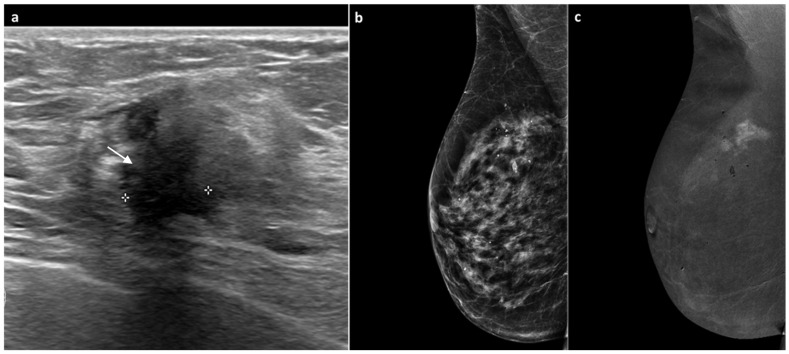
A 77-year-old woman, previously treated for DCIS of the right breast with partial mastectomy 11 years earlier with tenderness at the surgical scar and increased breast consistency. Ultrasound revealed heterogeneous echotexture of the surgical scar with a maximal thickness of 1 cm (white arrow, (a)). Mammography showed no significant changes or suspicious findings (BI-RADS 3) (b). Subsequent CEM demonstrated a marked, intense, multilobulated mass-type enhancement measuring approximately 1.8 cm (c). An ultrasound-guided biopsy diagnosed invasive lobular carcinoma, Luminal B subtype. Total mastectomy confirmed invasive lobular carcinoma with negative axillary lymph nodes.
